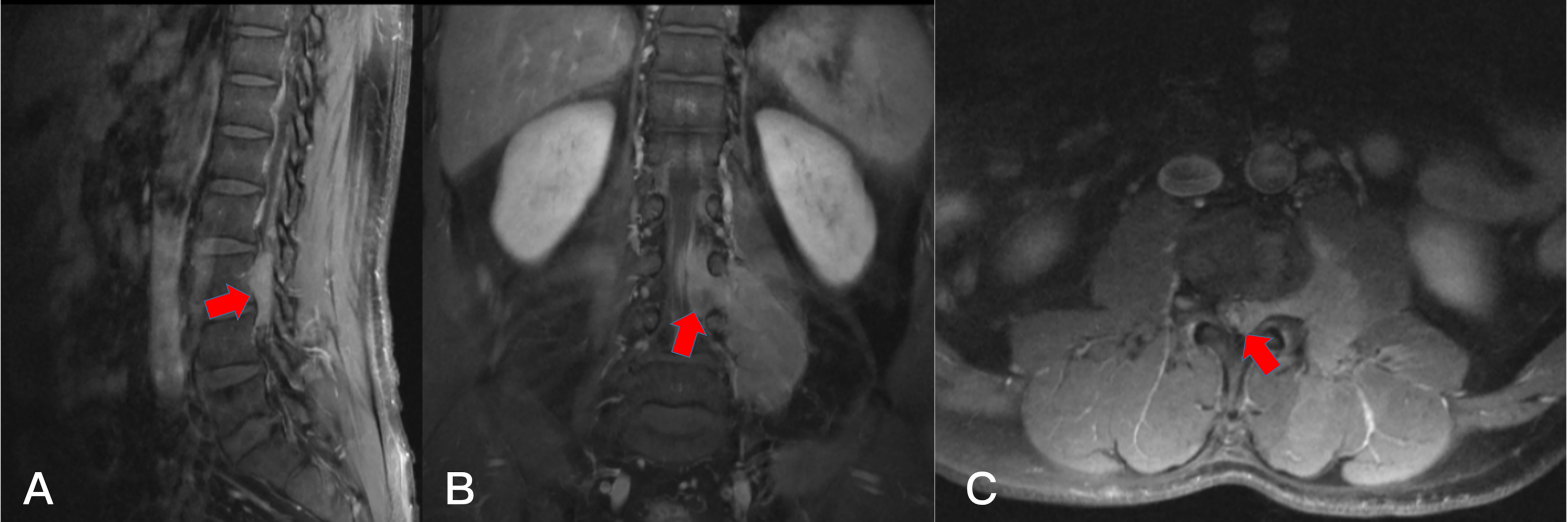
Preoperative T1-weighted enhanced MRI on sagittal (A) plane, coronal (B) plane, and axial (C) plane, revealing a dumbbell tumor (Eden II) in the left L3-4 foramen (red arrows).
